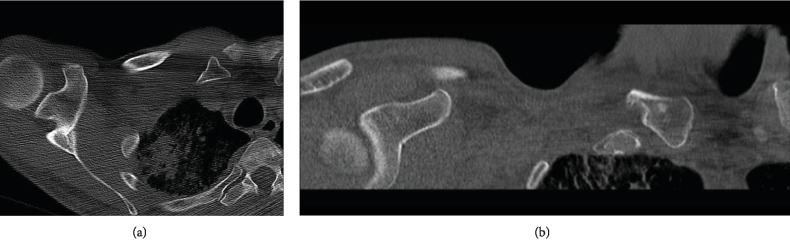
CT images five months after the initial injury showing a right medial clavicle fracture nonunion (a) with sclerotic changes of the medial clavicle fragment (b).
